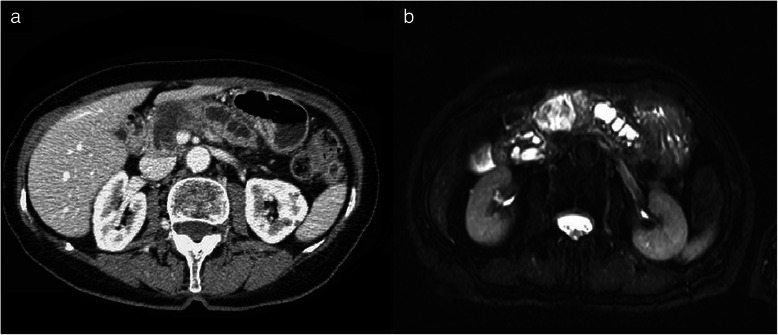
a Contrast enhanced CT scan demonstrates a complex pancreatic macrocystic mass involving the neck and the body of the pancreas with a peripheral solid portion. b Axial T2-weighted MRI shows the high-intensity central cystic portion of the mass with inner irregular septa and the peripheral less intense solid tissue determining main duct dilatation
